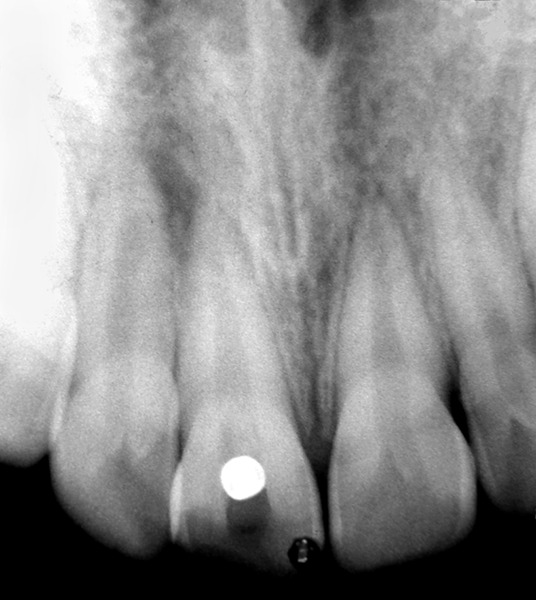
Preoperative radiograph showing the fractured right maxillary central incisor with a radiopaque foreign object
